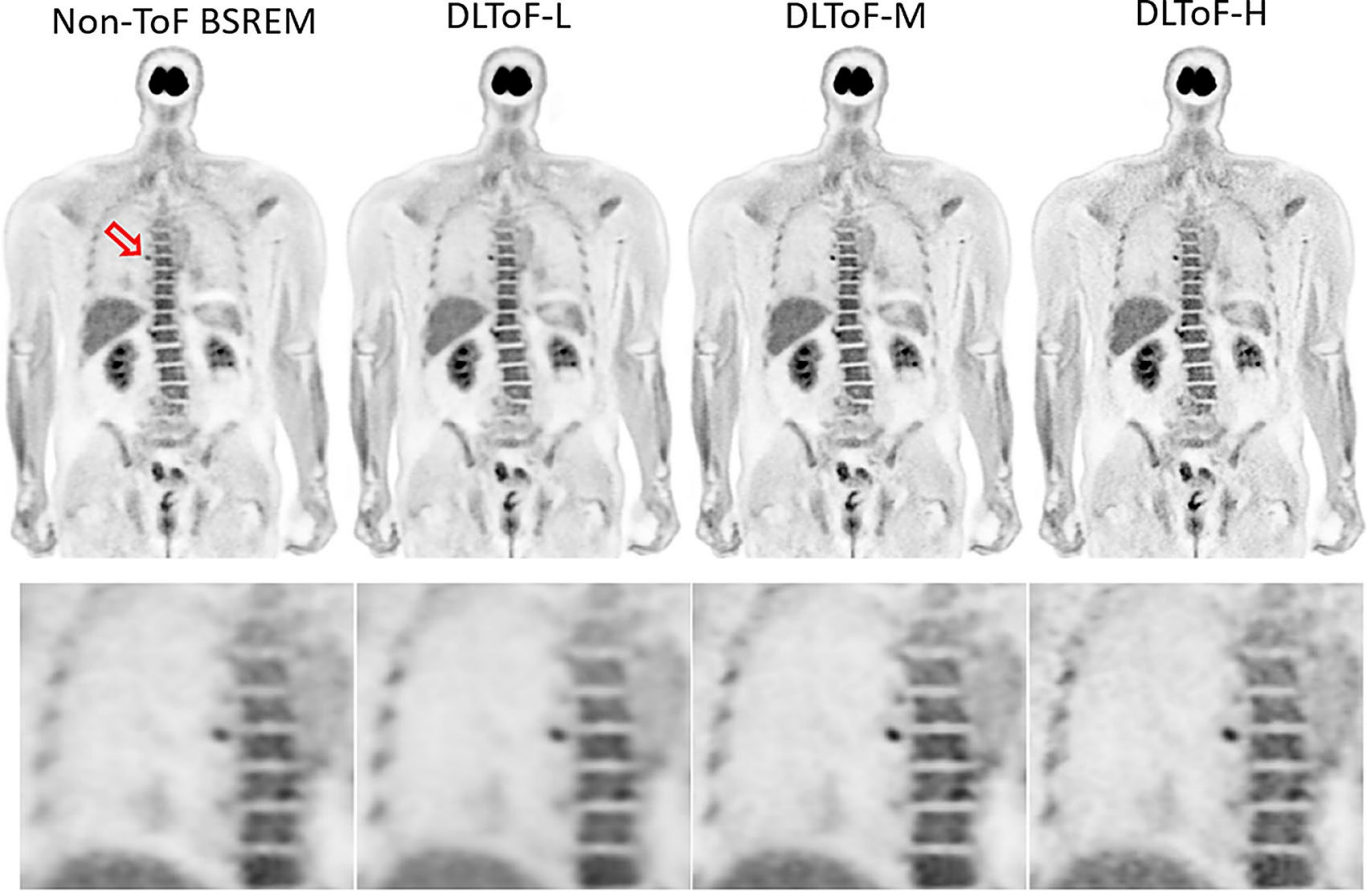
DL-ToF enhancement of a representative ^18^F-FDG test subject with a BMI of 26.3 kg/m^2^ with an injected activity of 346 MBq scanned on a GE Omni Legend PET/CT scanner. Display window: 0–5 SUV
